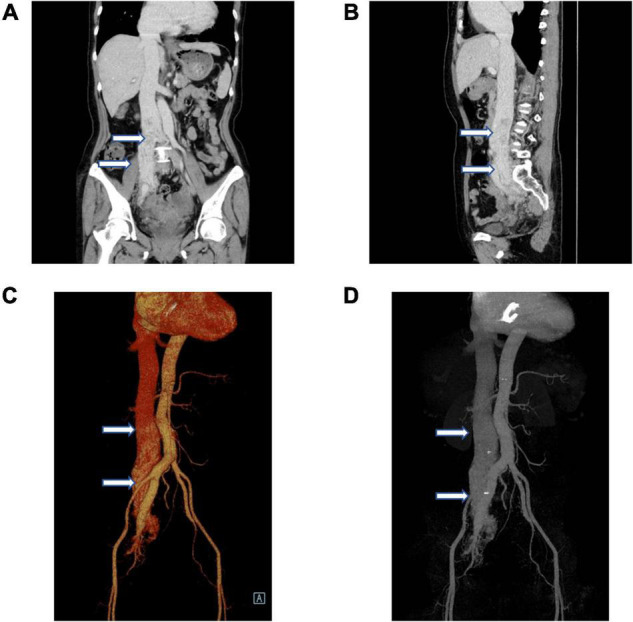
Vascular computer angiography (CTA) and 3D reconstruction show (A–D) the mass in the posterior wall of the uterine fundus extends to the pelvic veins, bilateral internal iliac veins, and inferior vena cava. The tumor did not invade the superior segment of the inferior vena cava and the heart (white arrows).
